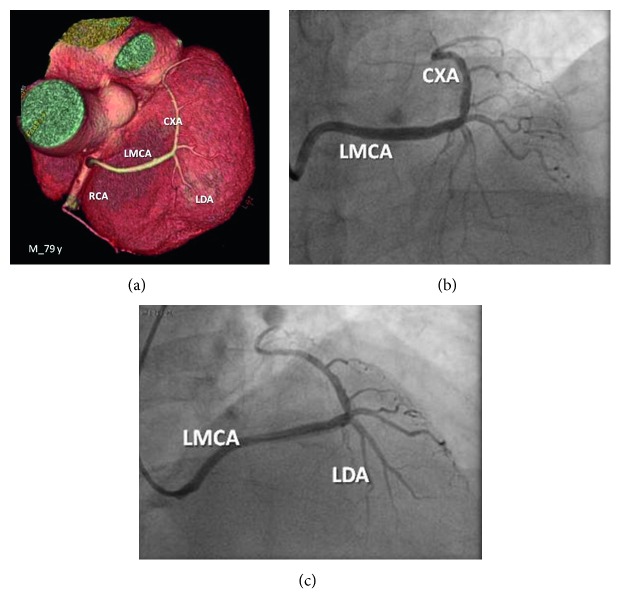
CT angiography and coronary angiography showing a long and trifurcated left main coronary artery with a prepulmonic course.
